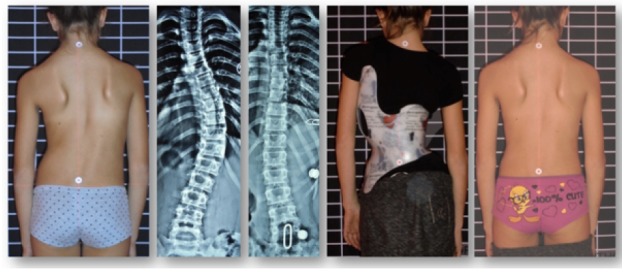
Gensingen Brace^®^ treatment for a single curve pattern showing an overcorrection in the x-ray and a slight cosmetic improvement after 6 weeks of brace wearing time. This Gensingen brace has been made in Greece (With kind permission by Nico Tournavitis, SBPRS, Thessaloniki, Athens, Nicosia).
